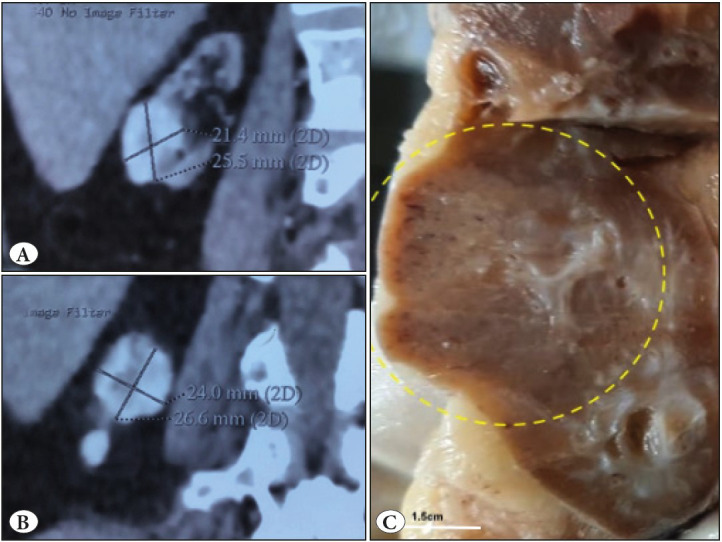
A, B) Contrast-enhanced CT abdomen showed a well-circumscribed tumour nodule in the upper pole in the background of a small kidney C) Cut surface shows a well-demarcated tan brown colored tumour measuring 3x2.8x2.5cm in the mid-third region.
